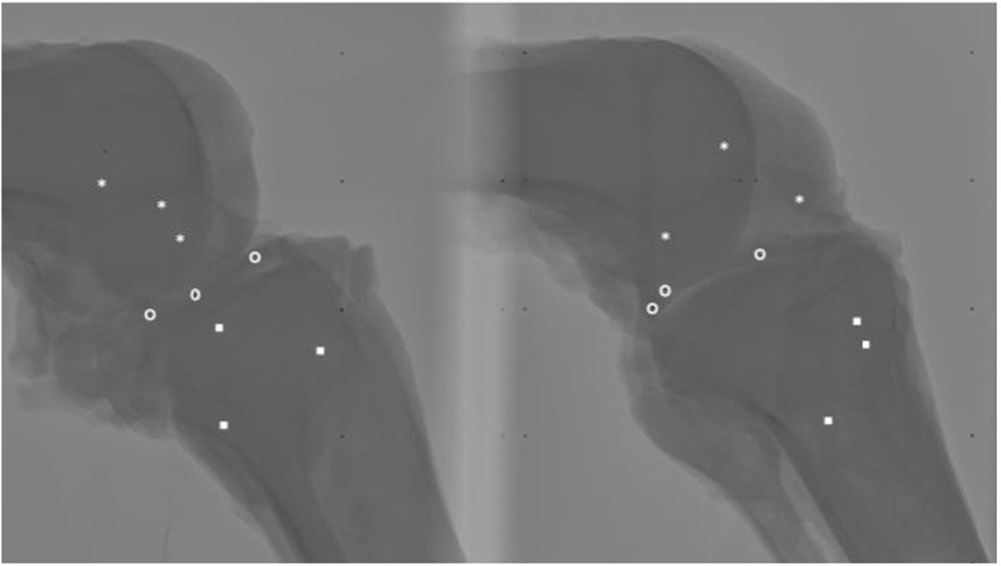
Example of a stereo Roentgen image, with beads detected in femur (*), meniscus (o) and tibia (■).
